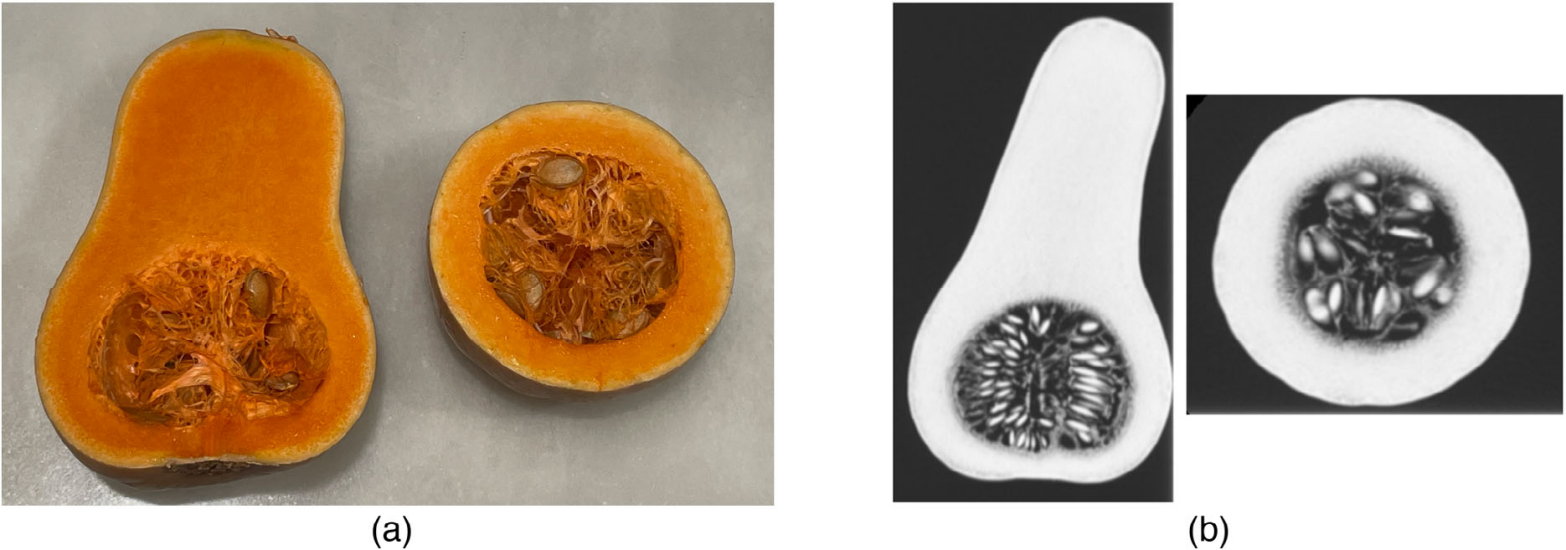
Photographs (a) of butternut squash shown in the lesson plan cut into segments to help students visualize coronal and axial orthogonal planes. Squash was compared to CT images (b) of a similar butternut squash.
